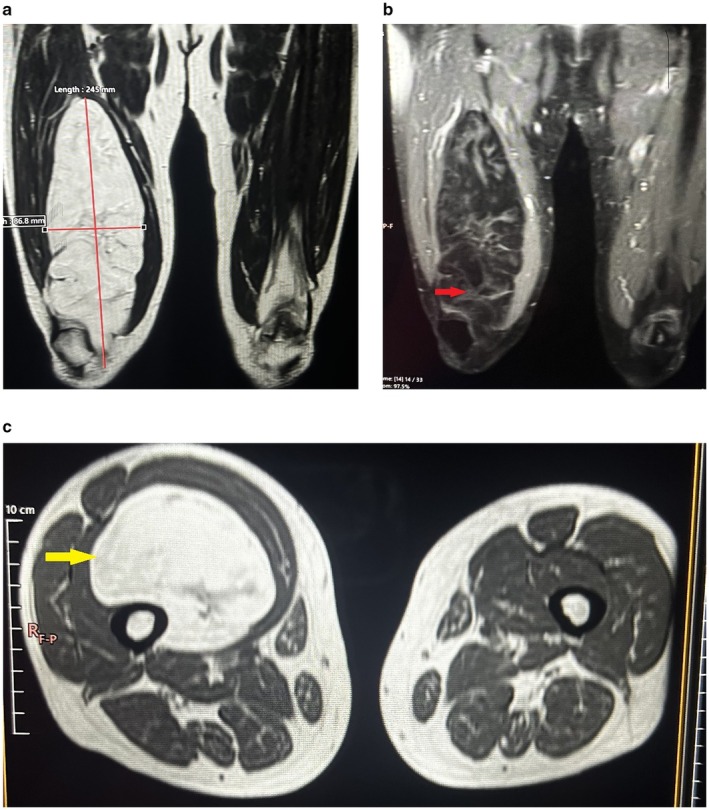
(a) Magnetic resonance imaging of the right thigh (coronal section) demonstrates a large, well‐defined, lobulated intramuscular mass located along the medial aspect of the thigh. The lesion measures approximately 24.5 cm in craniocaudal length and 8.6 cm in transverse diameter. The mass appears homogeneously hyperintense on T1‐weighted imaging, with signal characteristics identical to subcutaneous fat, consistent with a lipomatous lesion. (b) MRI right thigh coronal view with contrast showing no contrast enhancement with irregular septation of mass (red arrow). (c) MRI right thigh axial view T2‐weighted image showing well capsulated soft tissue mass at right thigh (yellow arrow).
